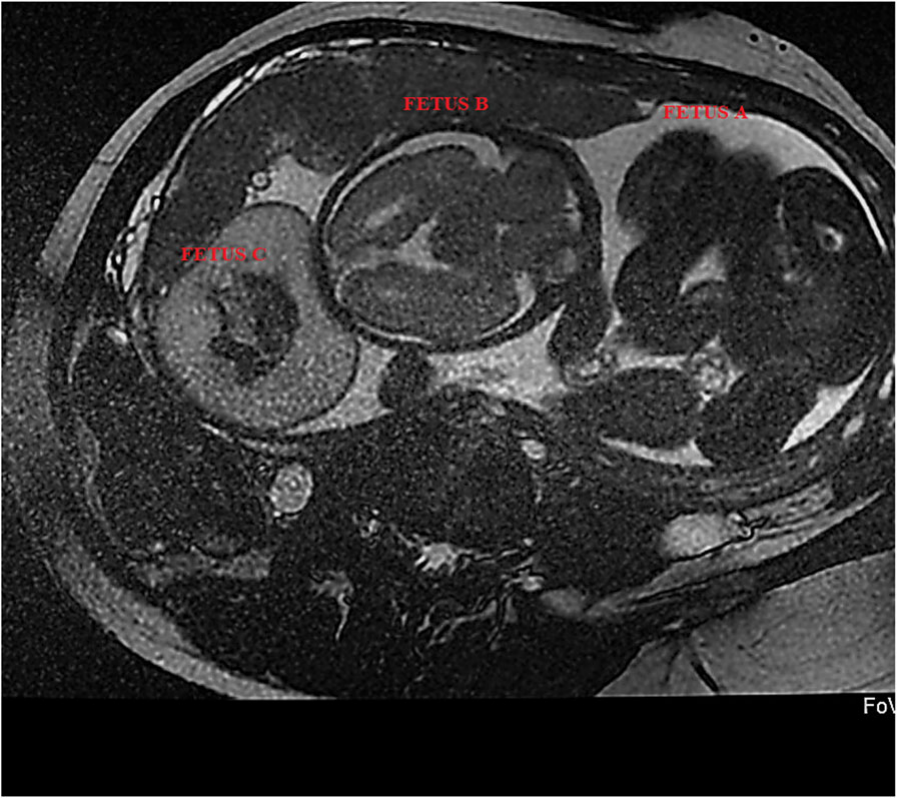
Acardiac fetus image by ultrasonography
